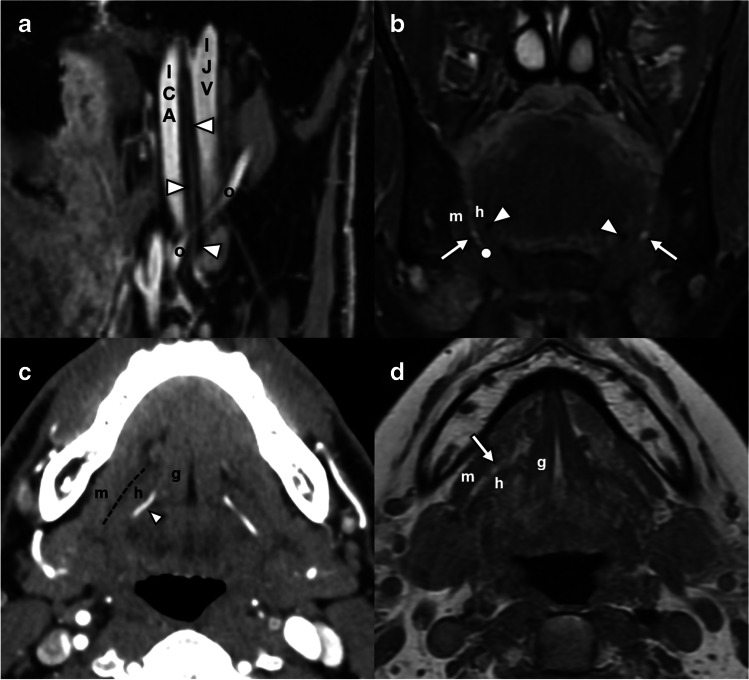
Imaging anatomy of the carotid and sublingual segments of the hypoglossal nerve. a Sagittal oblique volumetrically acquired T1FS sequence through the suprahyoid neck demonstrates the path of the HN (arrowheads) in the carotid sheath, between the internal carotid artery (ICA) and the internal jugular vein (IJV) before crossing the occipital artery (o) and turning anteriorly to enter the sublingual space. b Coronal T2FS sequence through the floor of mouth at the level of the angle of the mandible demonstrates the anticipated location of the hypoglossal nerve (dot) in the sublingual plane between mylohyoid (m) and hyoglossus (h) muscles and inferior to the submandibular duct (arrow). Note the lingual artery flow voids (arrowheads) deep to hyoglossus. c Axial contrast-enhanced CT angiogram demonstrates the plane of the hypoglossal nerve (dashed line) between the genioglossus (g), hyoglossus (h), and mylohyoid (m). Note how the lingual artery (arrowhead) lies deep to hyoglossus. d Axial T1W sequence through the floor of mouth demonstrates key landmarks including the genioglossus (g), hyoglossus (h), and mylohyoid (m) and the sublingual fat plane (arrowhead) through which the hypoglossal nerve traverses beneath the submandibular duct
